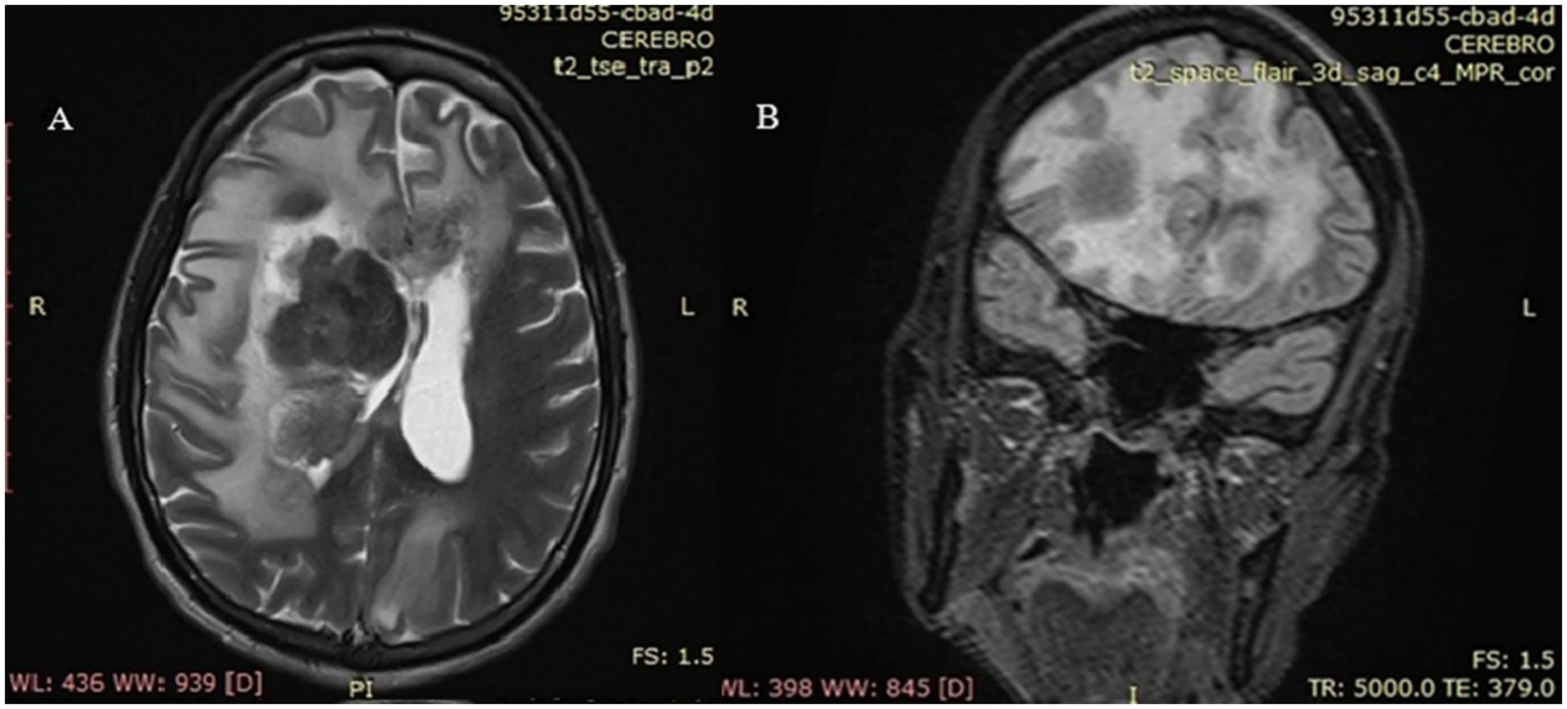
Brain magnetic resonance imaging. (A) Axial view – T2-weighted sequence; (B) Coronal view – FLAIR sequence: (A) A heterogeneous lesion is observed in the right cerebral hemisphere, centered in the lenticulocapsular region, with peripheral T2 hyperintense areas (edema) and a hypointense center, consistent with necrosis. There is collapse of the right frontal horn, displacement of the septum pellucidum, and compression of the ventricular system. No overt transtentorial or subfalcine herniation is evident in this section, although there is marked mass effect. (B) A centrally hypointense space-occupying lesion with necrosis, edema, and mass effect is noted. A hyperintense halo is seen in the right basal ganglia region, extending toward the internal capsule and adjacent thalamus, with irregular margins and surrounded by extensive vasogenic edema involving the right cerebral hemisphere. Compression of the frontal horn of the right lateral ventricle and mild leftward midline shift are identified. The perilesional hyperintense pattern on FLAIR suggests a lesion with central necrosis and fluid content, surrounded by edema. The axial T2-weighted image complements the FLAIR view, clearly demonstrating the cystic/necrotic structure with surrounding edema.
